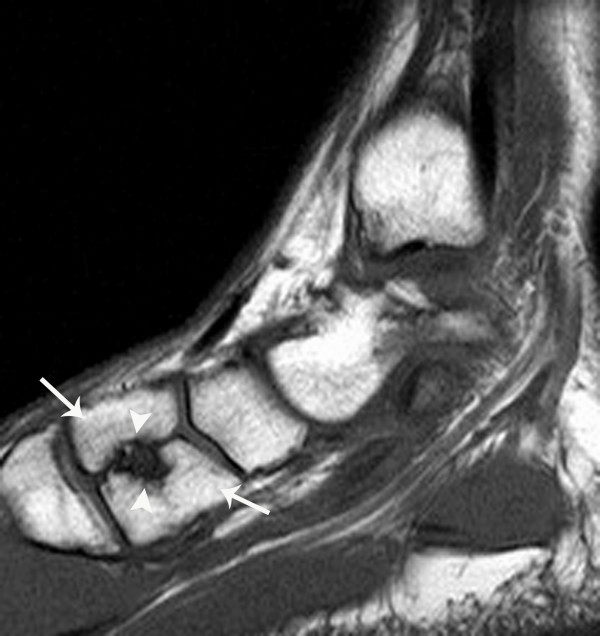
Sagittal T1 weighted spin echo (TR/TE = 700/9 ms) MR image of a partially bipartite medial cuneiform with early osteoarthritis of case #4. The cuneiform clearly includes two morphologic fragments (arrows) and there are erosive changes (arrowheads) about the cleavage plane suggesting partial fusion and some instability or motion between fragments.
